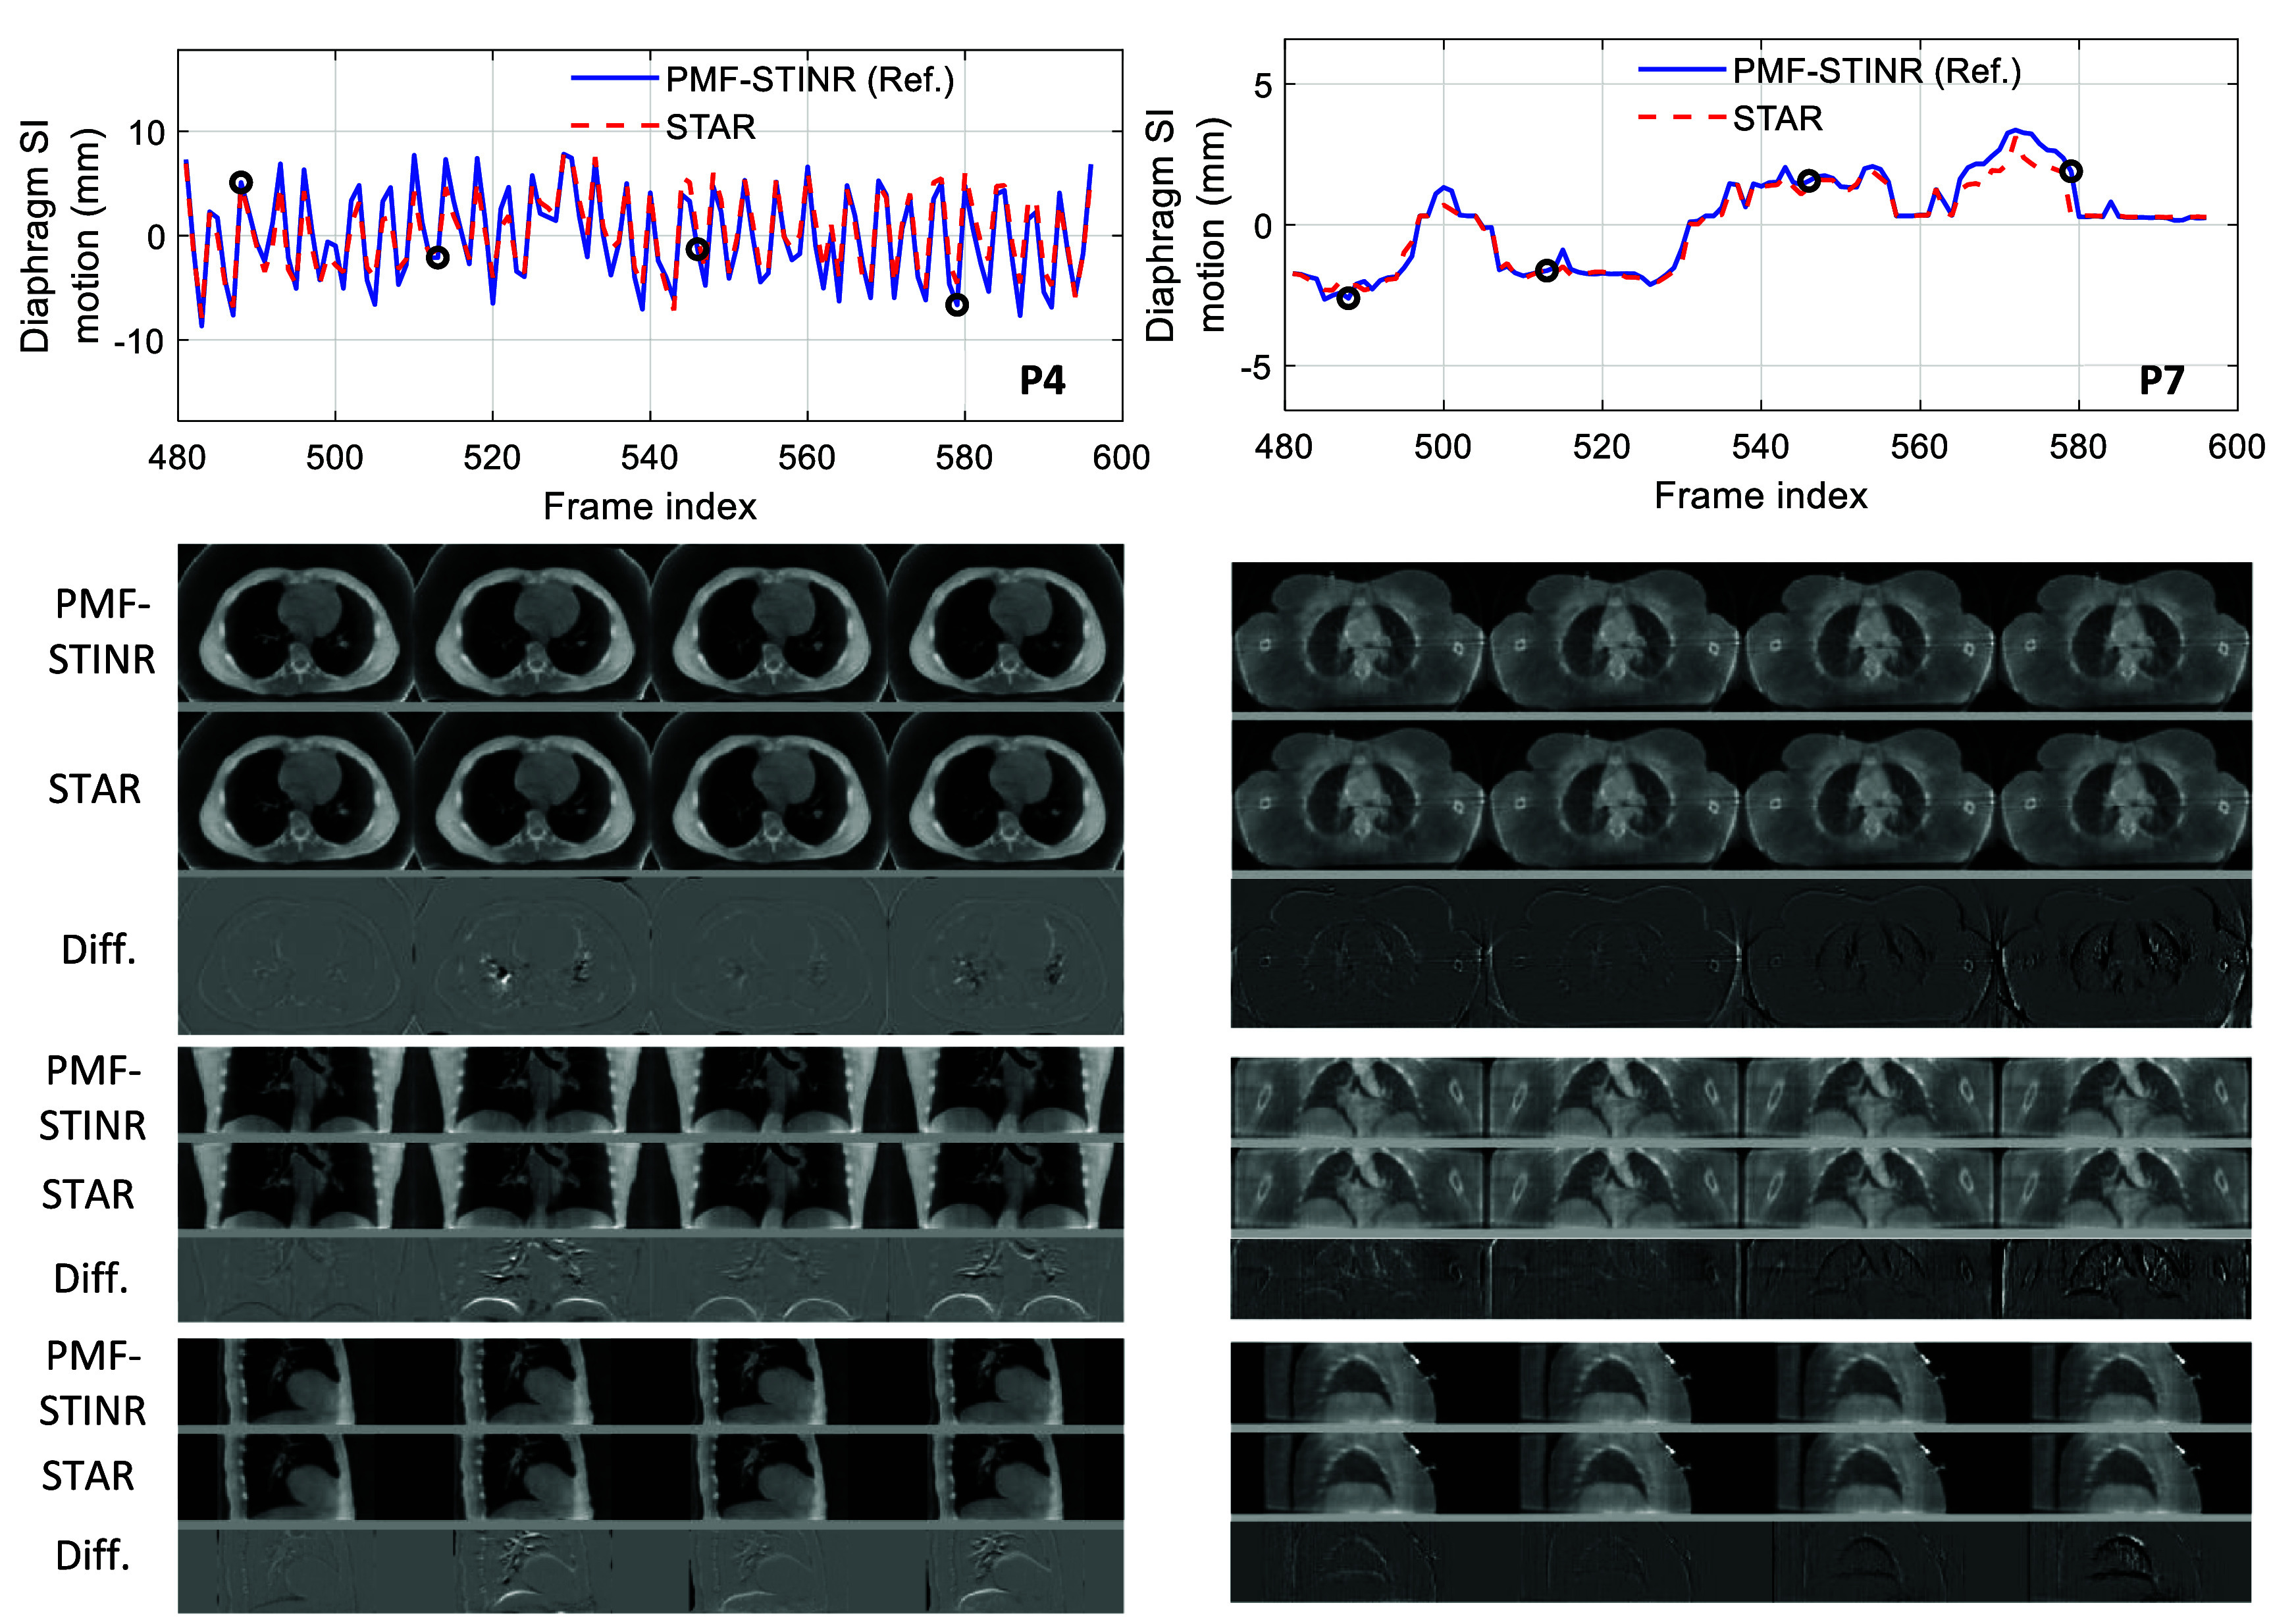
Motion profile and sectional image comparisons of the patient study for two example patients: P4 (left) and P7 (right). The top panel shows the diaphragm motion profiles in the SI direction. The second, third, and fourth panels show the axial, coronal, and sagittal images at four selected time indices, marked by the black circle on the motion profiles. The third row of these three panels shows the difference images between the STAR CBCTs and the dynamic CBCTs (based on PMF-STINR). The display window for P4 is [−1000, 820] HU for the sectional images and [−1360, −640] HU for the difference images. The display window for P7 is [−1000, −170] HU for the sectional images and [−1020, −950] HU for the difference images.
